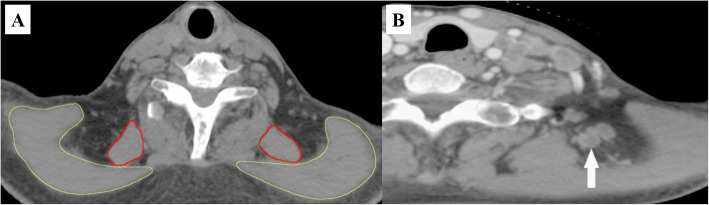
a. The PLV region in the CT scan. The PLV region is between the trapezius muscle and the scapular levator. The yellow line presents the trapezius, and the red line presents the levator scapulae. b. Metastatic lymph nodes in the PLV region. A typical picture of the metastatic lymph nodes in the PLV region is shown. The white arrows indicate the metastatic lymph nodes
